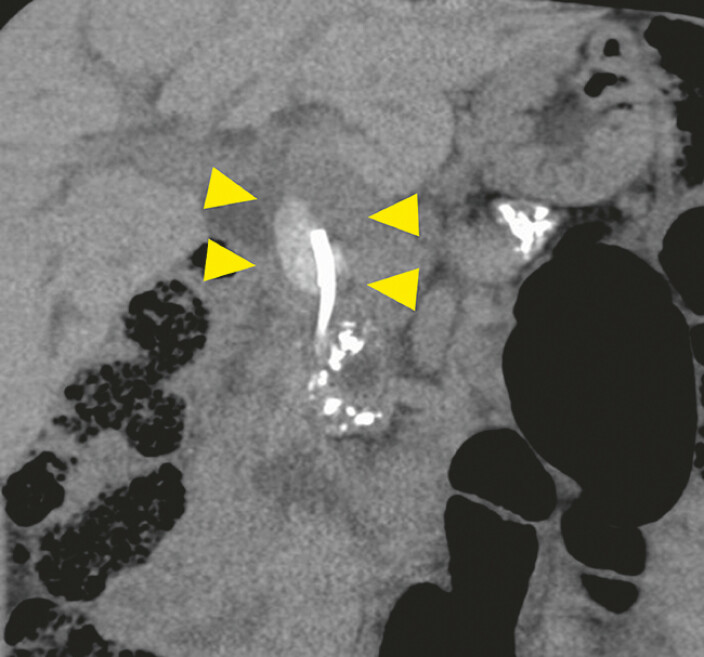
Computed tomography of a patient with chronic pancreatitis revealed a retained stent in the common bile duct with a large integrated stone (arrow).
